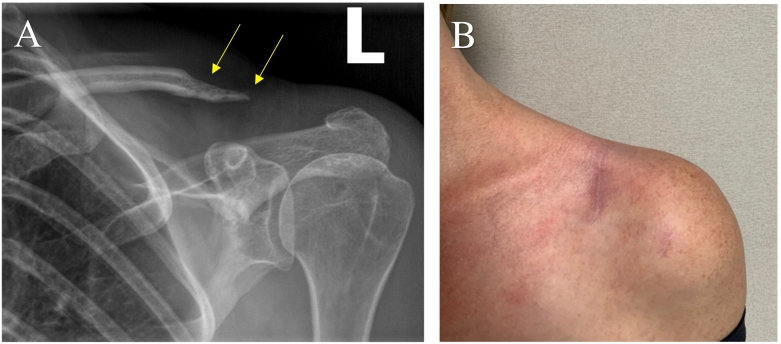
Four month anteroposterior follow-up radiograph (Image A) showing eventual cessation and stability of osteolysis (yellow arrows) while the patient remains asymptomatic with no functional deficits. Clinical photo (Image B) demonstrating no obvious clinical deformity with healed surgical incisions. (For interpretation of the references to colour in this figure legend, the reader is referred to the web version of this article.)
